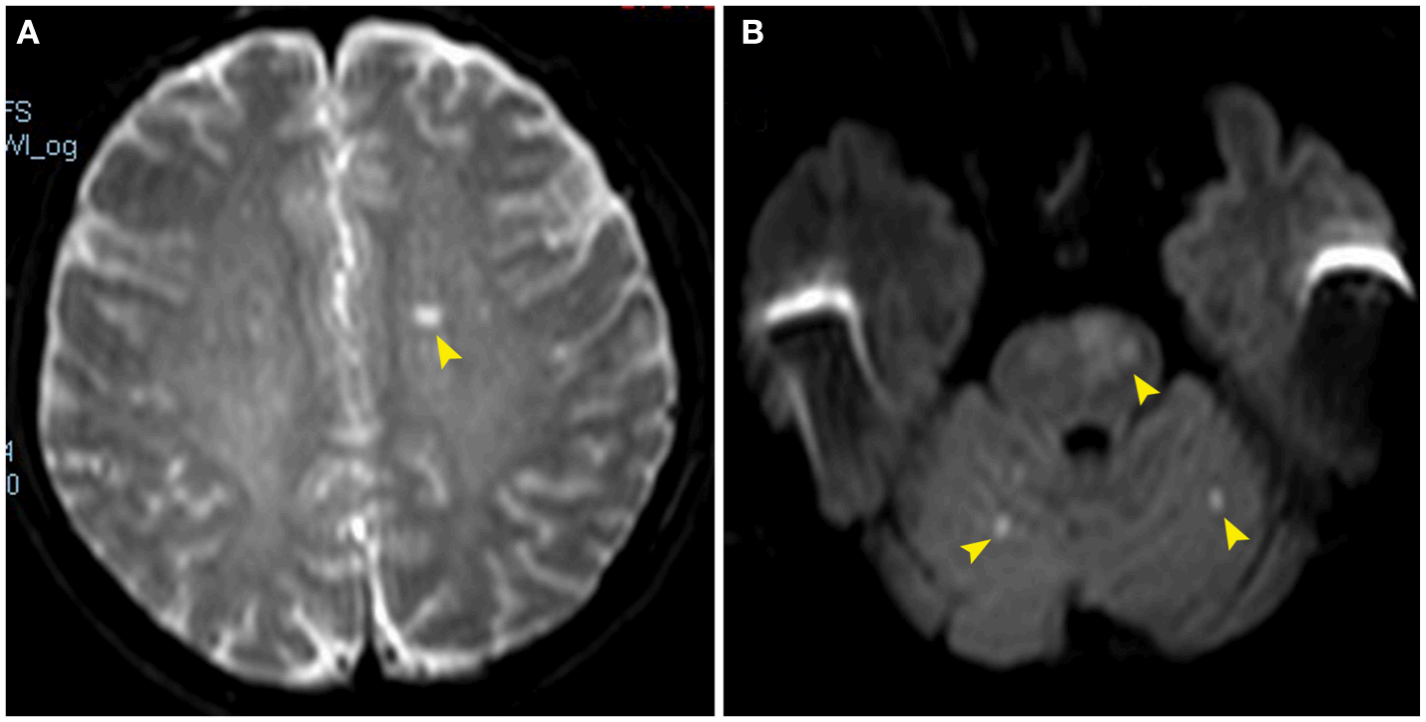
Diffusion-weighted magnetic resonance images showing acute infarcts involving the left corona radiate (A, arrowhead), bilateral cerebellar hemispheres and left pons (B, arrowheads).
